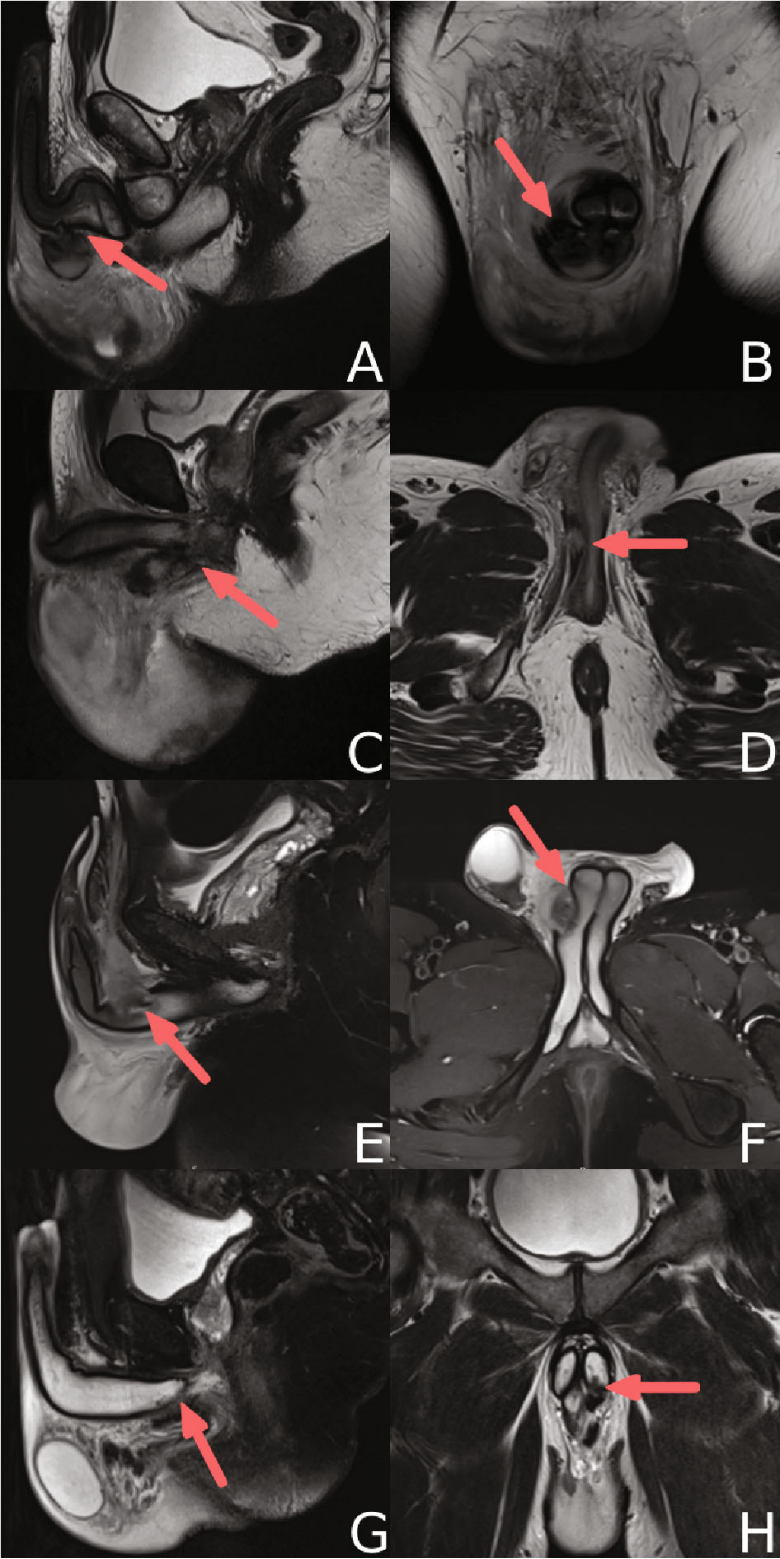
Penile MRI slices from admission. Red arrow indicates location of penile fracture. Patient A: T1-weighted MRI sagittal (A) and coronal (B). Patient B: T1-weighted MRI sagittal (C) and axial (D). Patient C: T2-weighted MRI sagittal (E) and axial (F). Patient D: T2-weighted MRI sagittal (G) and coronal (H).
